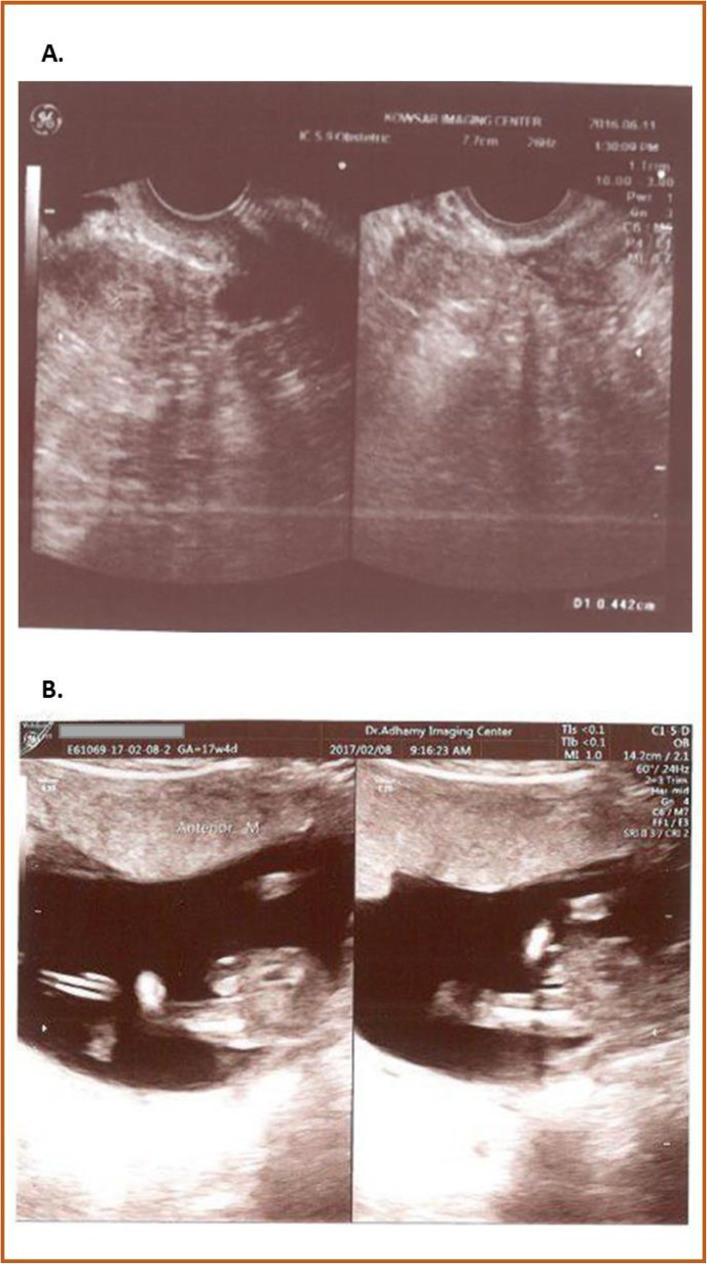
a Ultrasound of patient’s uterus at 31 years of age after Drospil treatment. Uterus measures 65 × 28 mm. b Pregnancy ultrasound at 17 + 4 weeks
